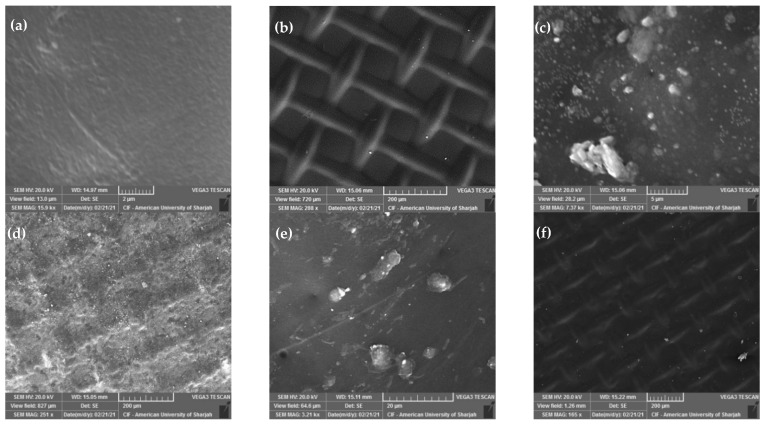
The effect of using ultrasound on water flux through a forward osmosis membrane during desalination of synthetic seawater. SEM images of the original membrane (a) active layer and (b) support layer; the fouled membrane after the use of a low CFV with the ultrasound facing the support layer (Case 0.25-CC-SL with NaCl draw solution) (c) active layer and (d) support layer; and the fouled membrane after the use of a high CFV with the ultrasound facing the active layer (Case 1.0-CC-AL with MgCl_2_ draw solution) (e) active layer and (f) support layer [144].
